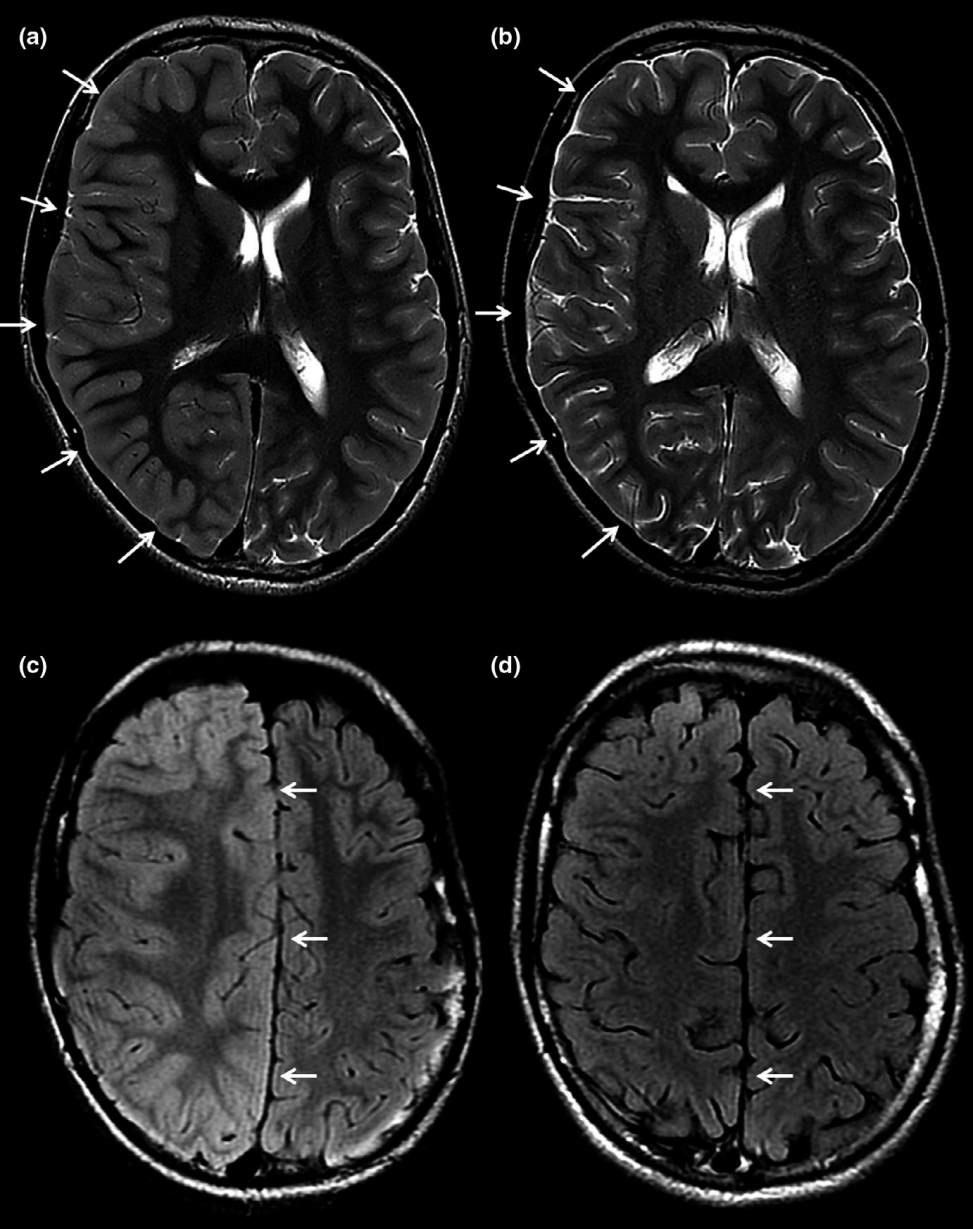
Axial T2‐weighted (a) and T2 FLAIR (b) MR images of a patient a few hours after motor seizures affecting the left side of the body and follow‐up MR images four months later (c, d). The axial images at the level of the lateral ventricles (a) and centrum semiovale (b) show diffuse swelling of the cortex of the right cerebral hemisphere with obliteration of the sulci (arrows in a) and a midline shift due to the mass effect (arrows in b). The follow‐up images at the same brain levels demonstrate normalization of the cortical swelling and midline shift (arrows in c and d)
